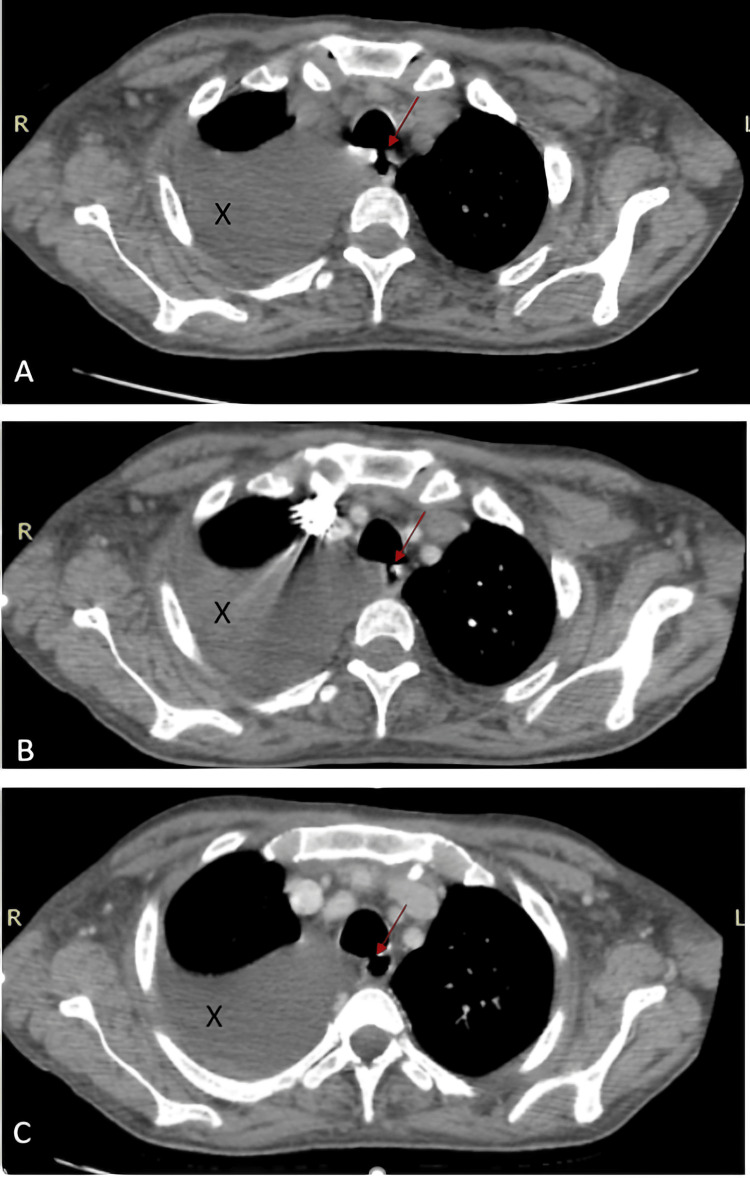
Axial CT scan images without contrast (A), in arterial phase (B), and venous phase (C) showing the TEF (red arrow) and pleural effusion (black X). TEF: tracheoesophageal fistula
